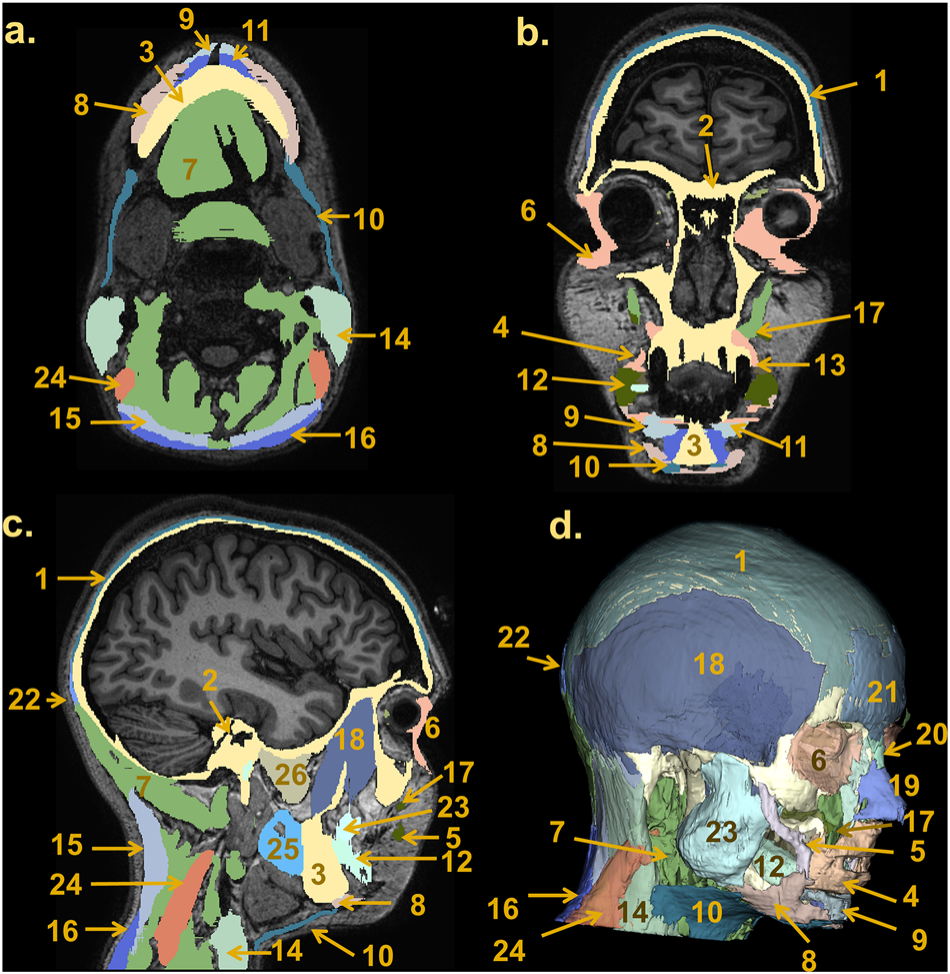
Segmentation of muscles. Axial (a), coronal (b) and sagittal (c) views of the individual and general muscle labels in the T1-weighted dataset. (d) Right anterior oblique view of the 3D reconstruction of the muscles. Legend of the structures: 1 Galea aponeurotica (tendon), 2 Skull, 3 Mandible, 4 Orbicularis oris, 5 Zygomaticus major, 6 Orbicularis oculi, 7 Muscles (general), 8 Depressor anguli oris, 9 Depressor labii inferioris, 10 Platysma, 11 Mentalis, 12 Buccinator, 13 Risorius, 14 Sternocleidomastoid, 15 Splenius capitis, 16 Trapezius, 17 Zygomaticus minor, 18 Temporalis & temporoparietalis, 19 Nasalis, 20 Procerus, 21 Occipitofrontalis frontal belly, 22 Occipitofrontalis occipital belly, 23 Masseter, 24 Levator scapulae, 25 Medial pterygoid, 26 Lateral pterygoid.
